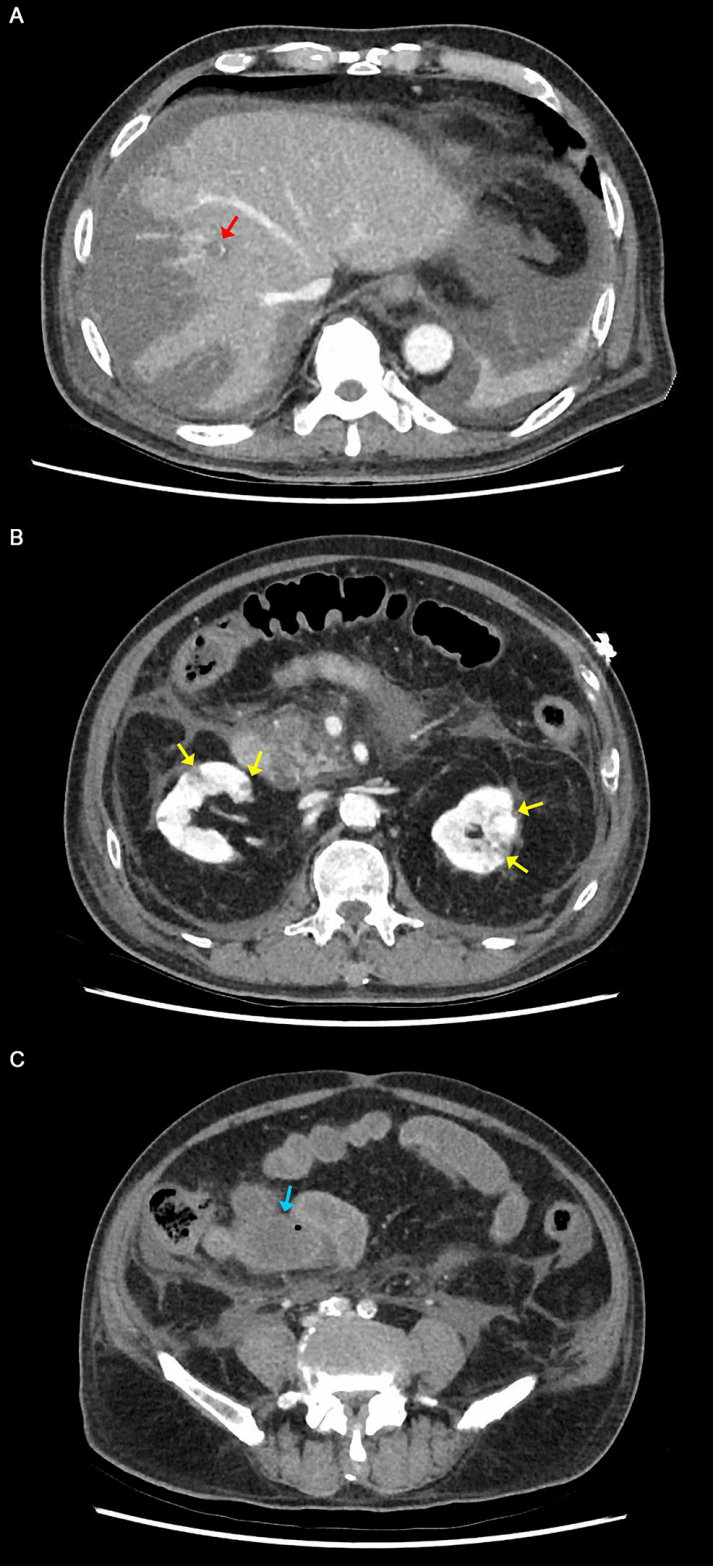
Contrast-enhanced CT in the portal venous phase done one day after admission (A) Thrombosis of the anterior segmental division of the distal right branch portal vein (red arrow) with multi-focal wedge-shaped hepatic infarcts in the right hepatic lobe. (B) Multiple peripheral wedge-shaped renal infarcts in bilateral kidneys (yellow arrows). (C) Relative hypoenhancement in the jejunal loops (blue arrow) indicating possible acute mesenteric ischaemia.
